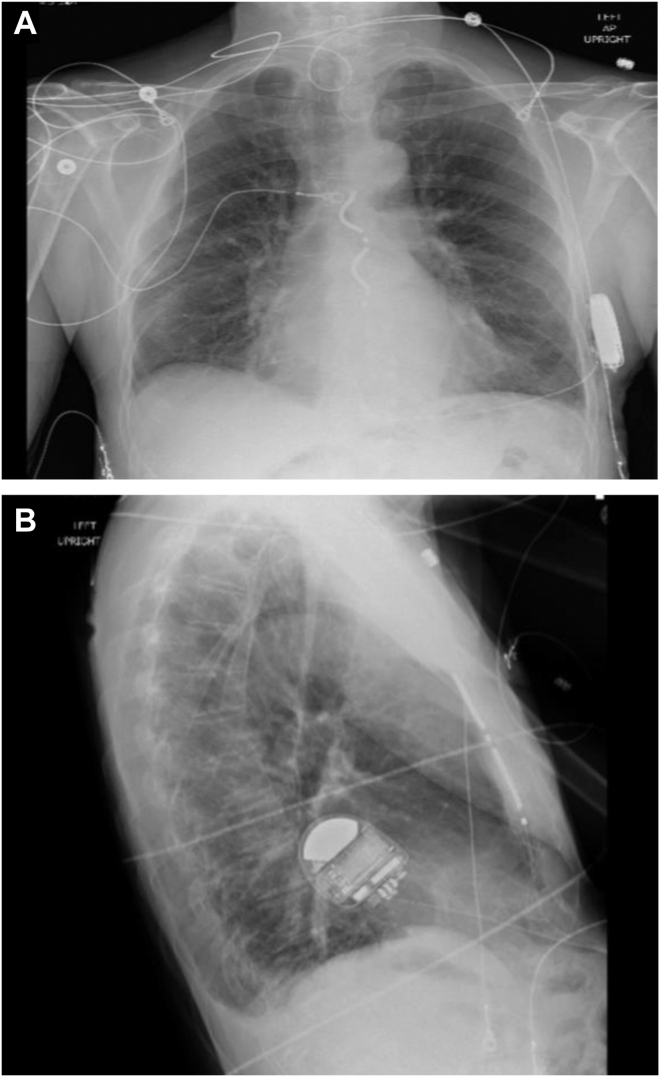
Follow-up chest radiograph following extravascular implantable cardioverter-defibrillator implantation demonstrating device positioning. A: Anterior view chest x ray. B: Lateral view chest x ray.
